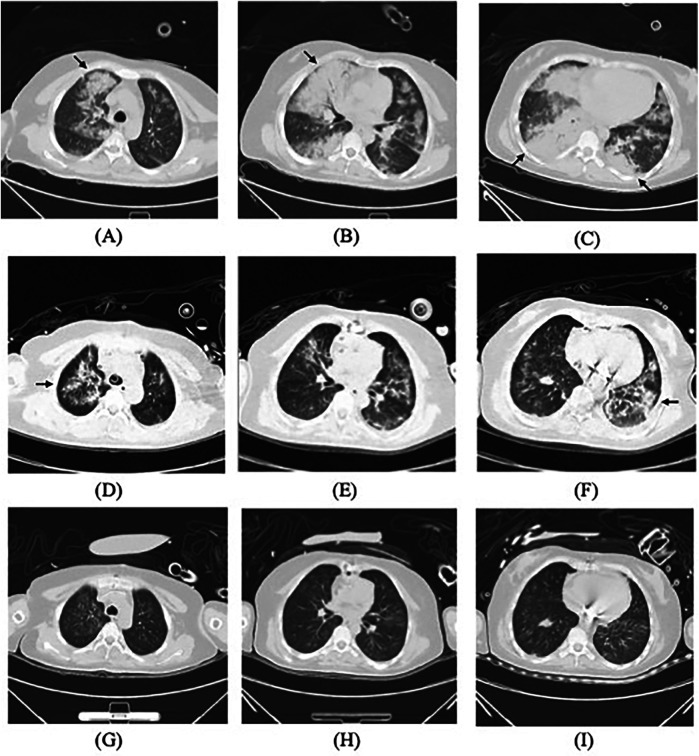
Lung CT scans: (A–C) on admission, (D–F) on the postoperative day 3, and (G–I) almost one week after initiating targeted treatment, demonstrating gradual resolution of pulmonary infiltrates (arrows).
